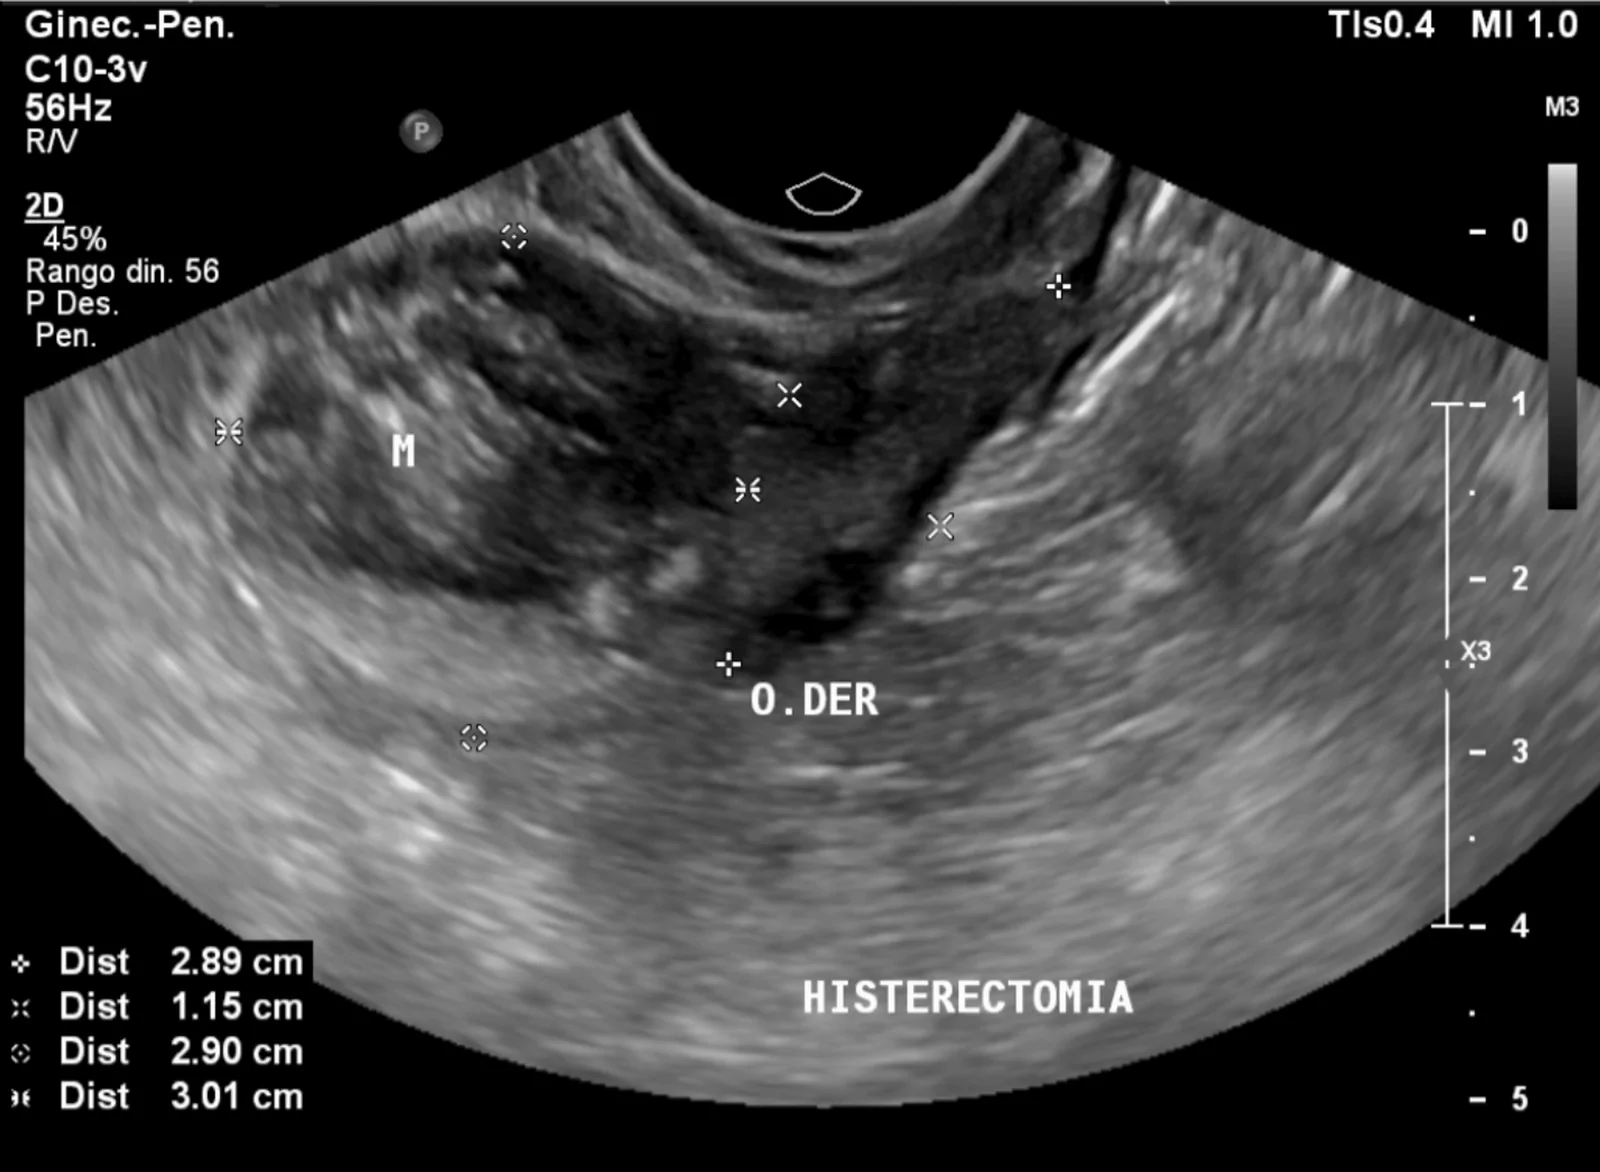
Initial endovaginal ultrasound showing a para-ovarian nodular image adjacent to the right ovary Endovaginal ultrasound demonstrating the right ovary (O.DER) with an adjacent heterogeneous, rounded para-ovarian nodular image with defined margins, measuring 2.89 × 2.90 × 3.01 cm, consistent with a dermoid cyst. The patient had previously undergone hysterectomy (noted on image).
